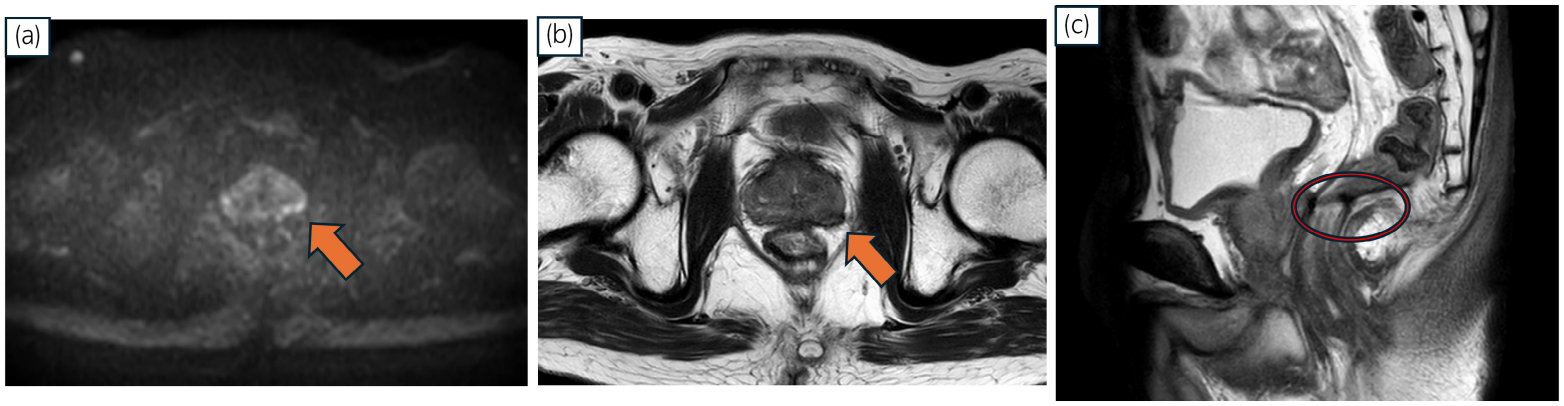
(a) Diffusion‐weighted and (b) T2‐weighted magnetic resonance images showed no malignancy at the time of recurrence. (c) Sagittal T2‐weighted image indicating the anastomosis site (red circle) behind the prostate, likely from the previous LAR.
